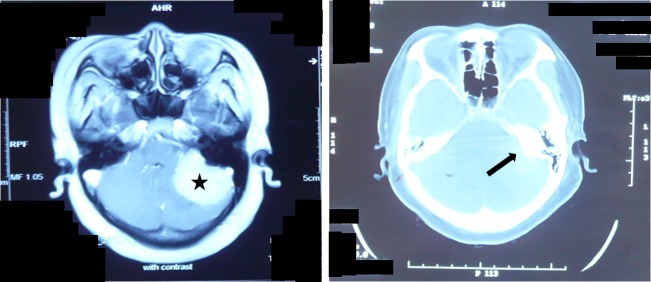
Reactive Thickening of the Petrous Bone MRI with contrast and CT demonstrating the location of the CPA meningioma and its petrous reactive changes in a 46-year-old female The CPA meningioma (star, left) was associated with a thickened petrous bone (arrow, right), but no dilated IAM. CPA: cerebellopontine angle; CT: computerized tomography; IAM: internal acoustic meatus; MRI: magnetic resonance imaging
